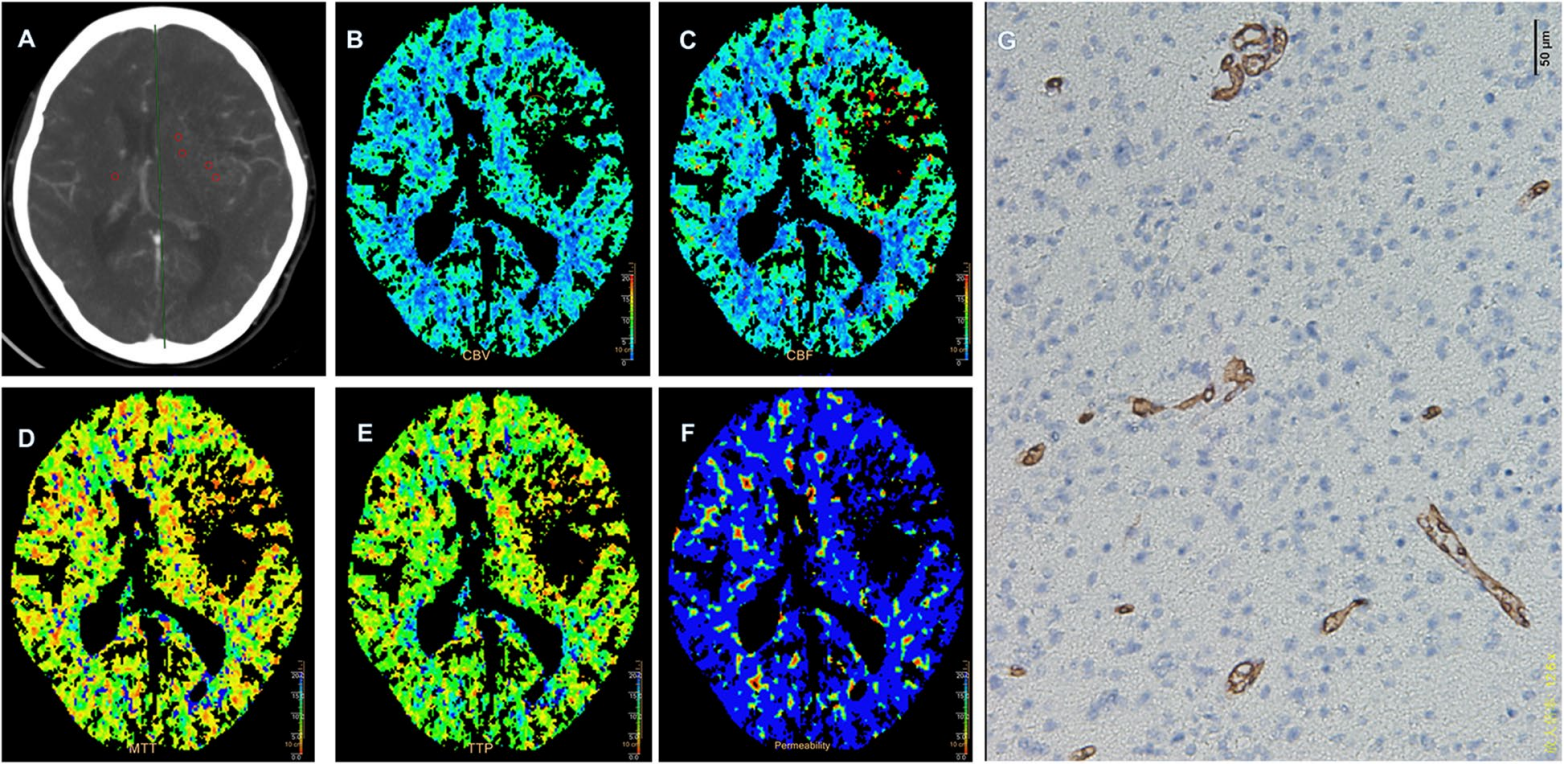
41-year-old woman with Left frontotemporal DA, WHO grade II, IDH-mut, TERT-wt, MGMT-un-met. A Transverse CT image and ROIs in red points. B PCT CBV map. C PCT CBF map. D MTT map. E TTP map. F PS map. E MVD map
